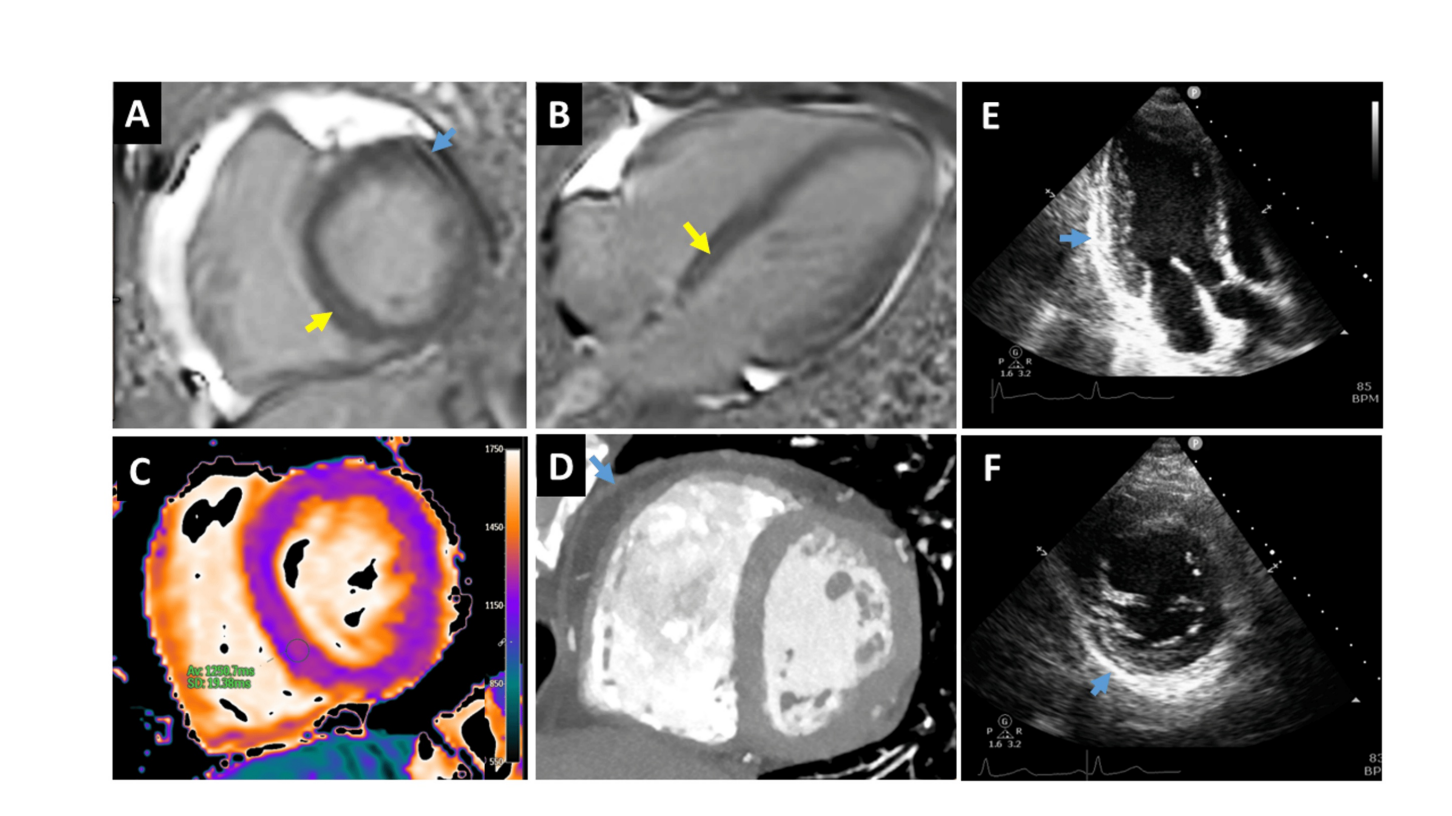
Multimodality images for the diagnosis of perimyocarditis CMR images demonstrate mid-wall late gadolinium enhancement (yellow arrows) in the basal septum (A, B) and diffusely increased T1 values (C) consistent with the diagnosis of myocarditis. CCTA (D) and echo images (E, F) demonstrate pericardial thickening (blue arrows), in addition to late gadolinium enhancement (LGE) of the pericardium (A), consistent with the diagnosis of pericarditis.  Of note, the CMR findings are less pronounced than more fulminant presentations found in the medical literature, which can exhibit subendocardial fibrosis and ventricular thrombi. This case represents an earlier stage presentation with increased T1 values and LGE, which are sufficient for the diagnosis given the clinical context. CCTA, coronary computed tomography angiography; CMR, cardiac magnetic resonance imaging
